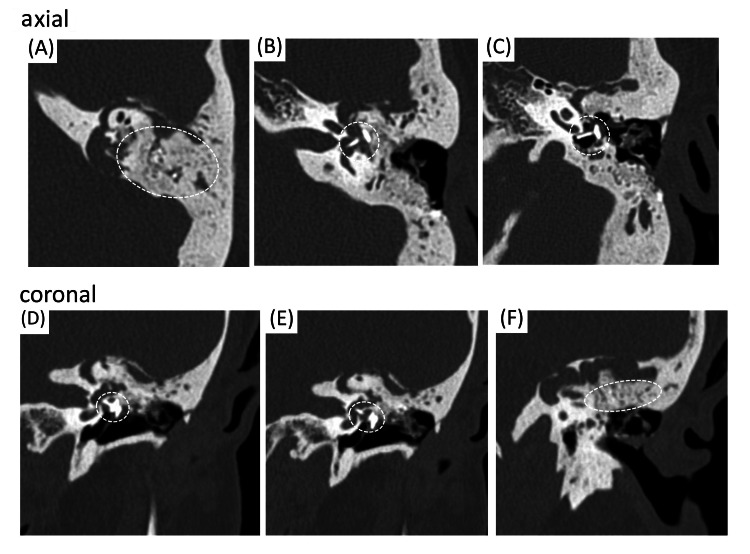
Findings from temporal bone CT A, F: Bone filling material (bone paté), presumably from the previous surgery, extending from the epitympanum to the semicircular canal area. B-E: Ossicular reconstruction material (Apaceram) from the previous procedure was found to be intruding into the vestibule.
